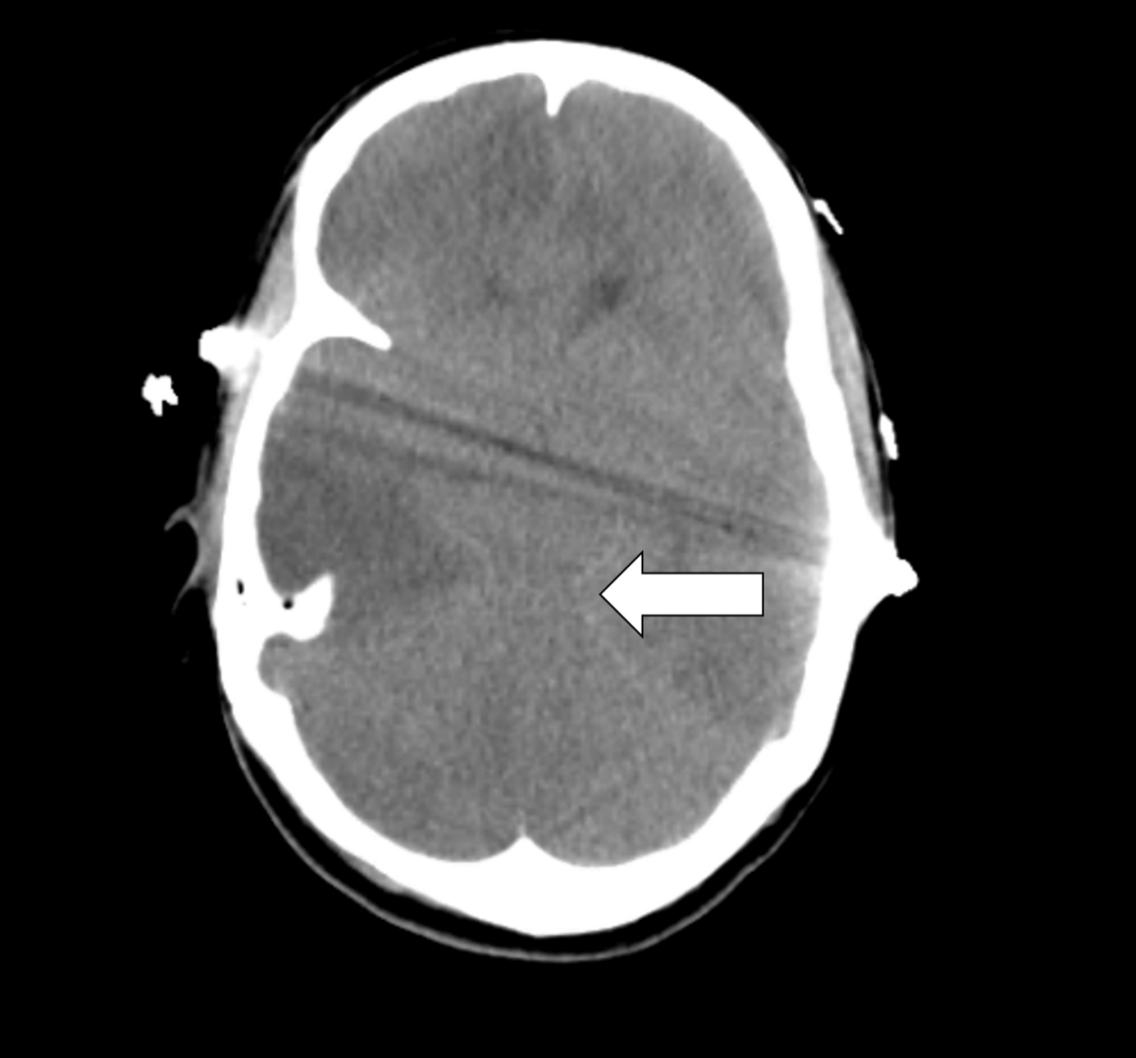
Post-intubation non-contrast computed tomography of the brain demonstrating loss of gray-white matter differentiation (arrow) with maintenance of perfusion of the thalami and deep gray matter concerning for global ischemic encephalopathy
